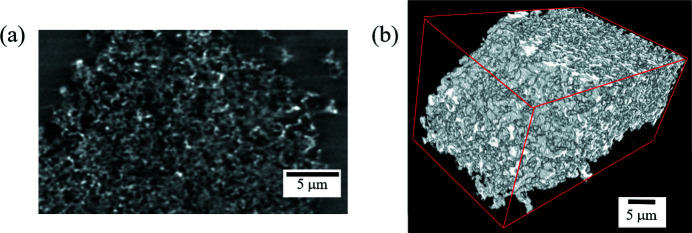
Nano-CT images of a cut MPL piece in dark phase-contrast mode. (*a*) Reconstructed CT image, and (*b*) 3D rendered image.
